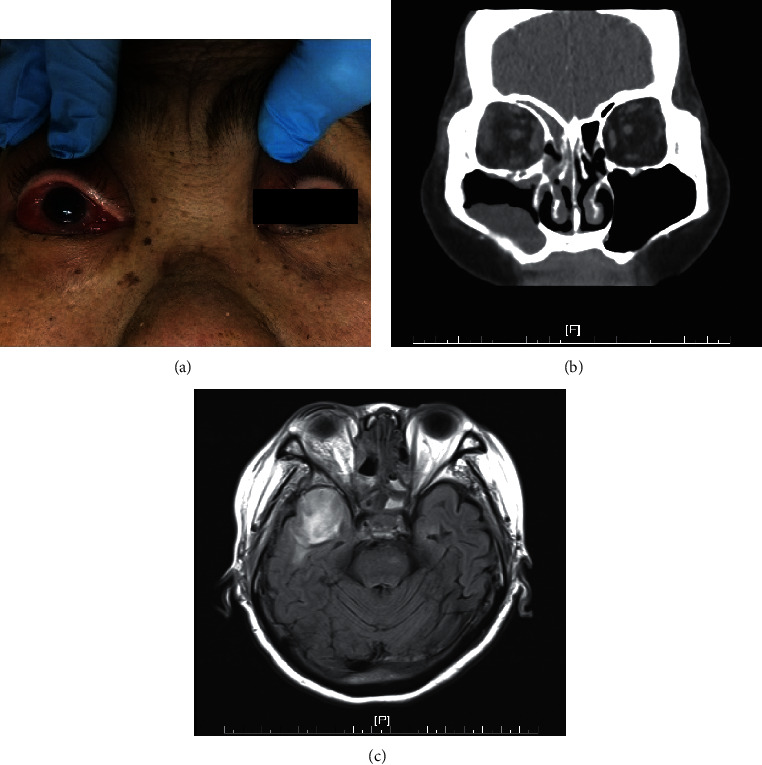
This was a 67-year-old female patient. Her pathological diagnosis was *Mucor* spp. She died 26 days after admission. (a) She presented as right eye blurry vision, limited extraocular movement, conjunctival injection and chemosis, and mild proptosis. (b) Computed tomography revealed orbital infiltration and subperiosteal abscess. Fluid accumulation over the frontal, ethmoid, and maxillary sinuses was seen. (c) Magnetic resonance imaging showed ipsilateral brain edema over the temporal region. This was taken 18 days after the CT image (b).
